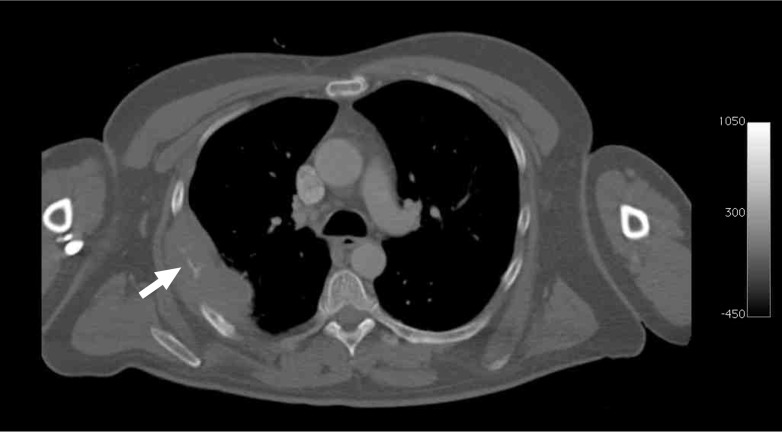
Example of tumor-induced cortical destruction at CT in a 49-year-old man with newly diagnosed DLBCL. Axial CT shows focal destruction of the right fifth rib (*arrow*) with surrounding soft tissue mass
